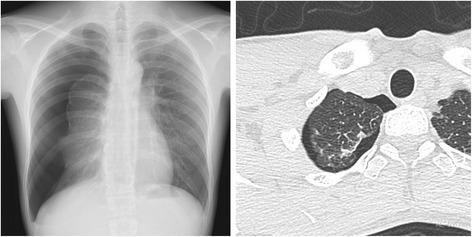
a Chest X-ray at the initial presentation revealed a right-sided pneumothorax. b Chest computed tomography demonstrated a few tiny blebs on the apex
